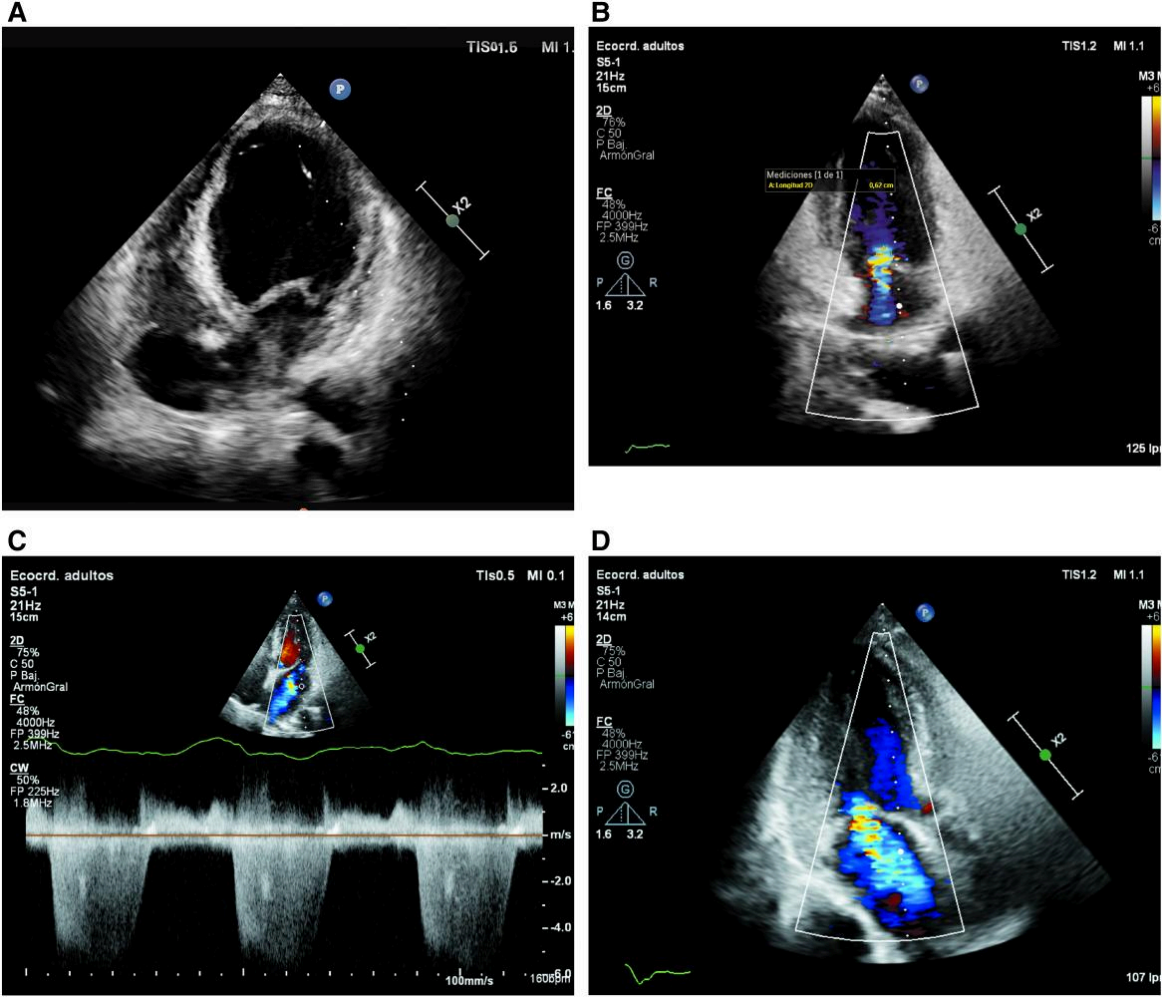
Moderate–severe mitral regurgitation. (*A*) Four-chamber view showing thickening of the mitral valve secondary to carcinoid disease. (*B*) Two-chamber view showing mitral regurgitation with a vena contracta measuring 6 mm. (*C*) Continuous-wave Doppler of mitral regurgitation. (*D*) Doppler image of mitral regurgitation in a three-chamber view.
